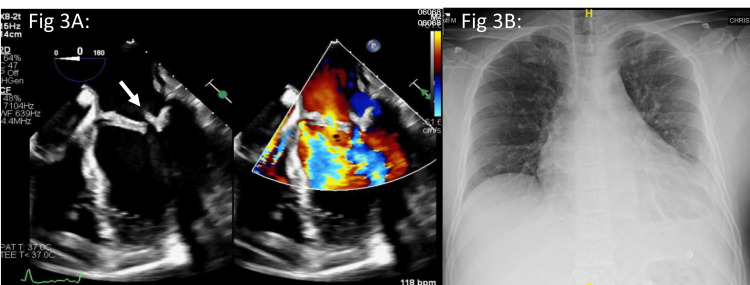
Case 3: Transesophageal echo and chest X-ray Transoesophageal echo (3A) showing an echogenic nodule at the tip of the posterior mitral leaflet on the atrial aspect (white arrow). Chest X-ray (3B) showing cardiomegaly and mild left pleural effusion.
